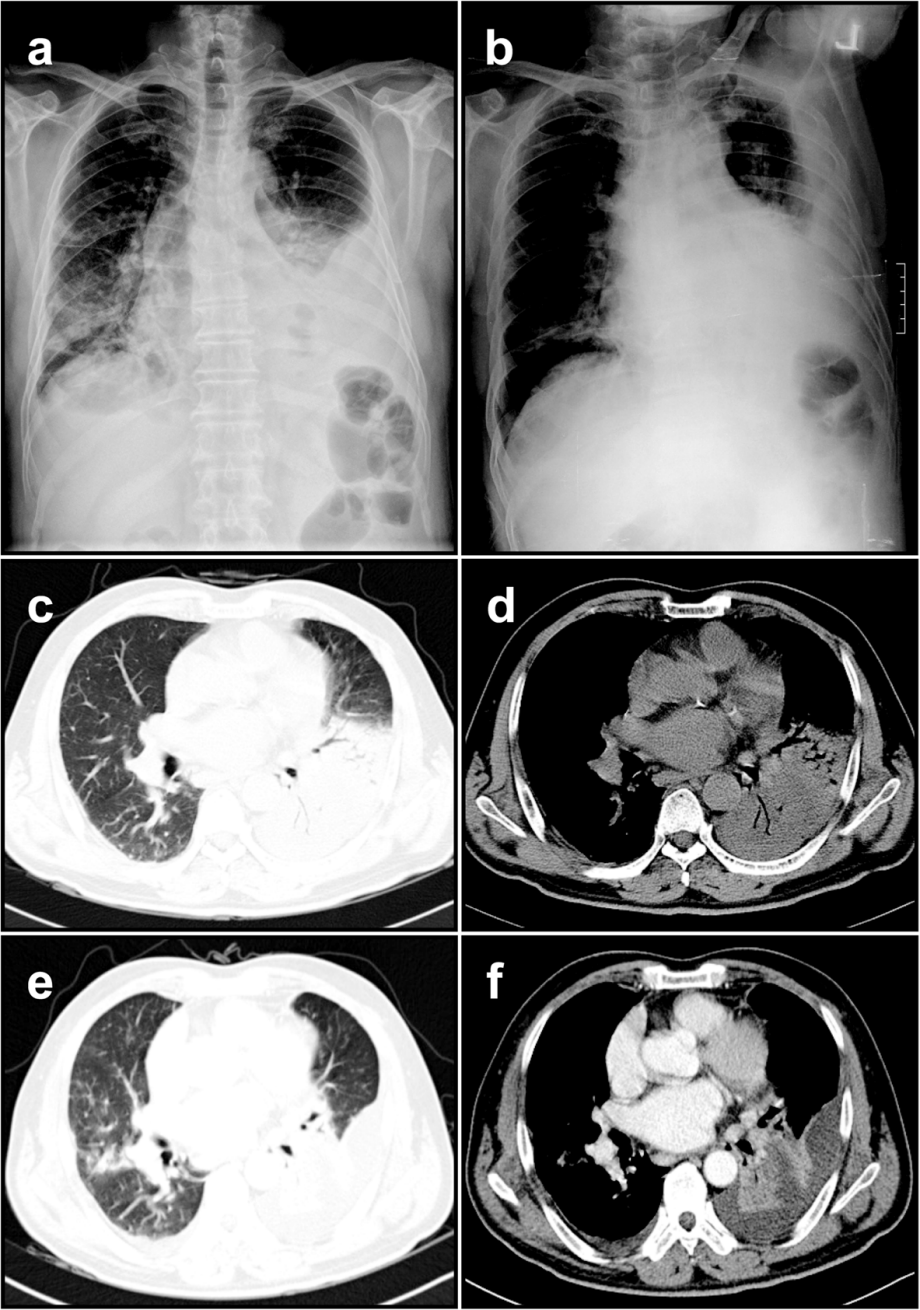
Chest X-ray and chest computed tomography (CT) findings in *M. lentiflavum*-infected patient. Chest Radiography (a; posteroanterior view, b; left decubitus view) shows consolidative lesion in the left lower lobe with fluid shifting sign. Chest CT scan revealed that solid consolidative lesion occupied the left lower lobe with air-bronchogram (c and d). Despite of the antibiotics therapy, the radiologic features were aggravated showing abscess formation in the left lower lobe, accompanying left pleural effusion and newly developed pneumonic infiltrative lesion on the right middle lobe (e and f)
